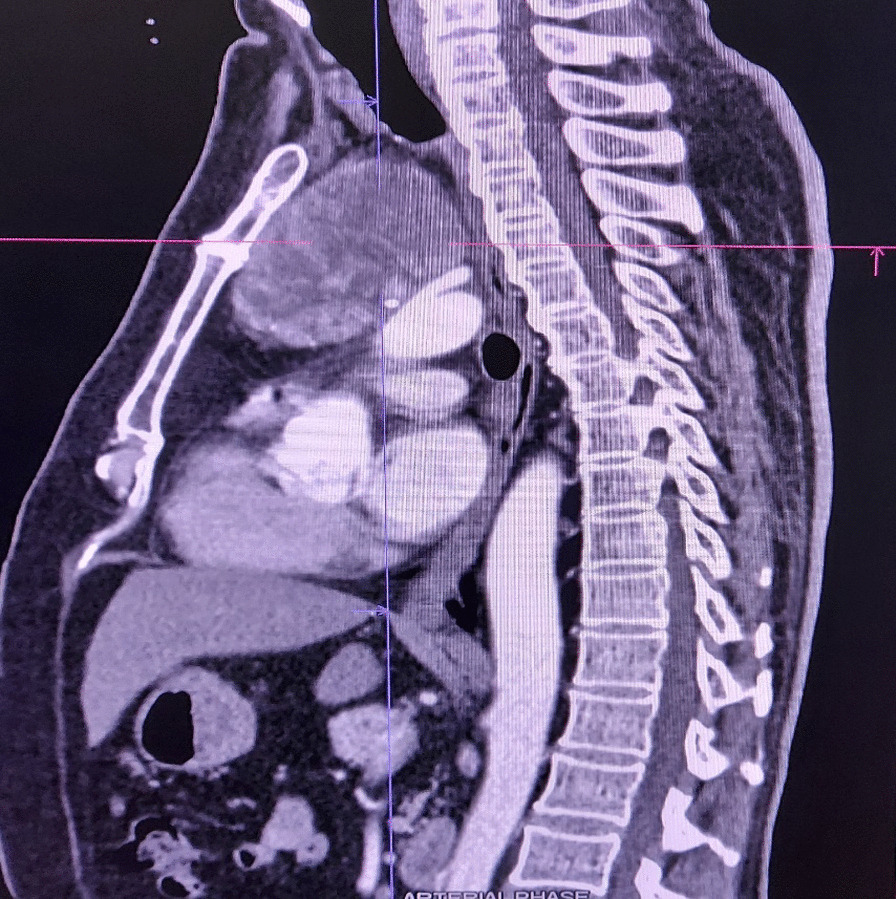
(CT scan revealing a mass with central necrosis and peripheral calcifications with normal looking thyroid gland)
